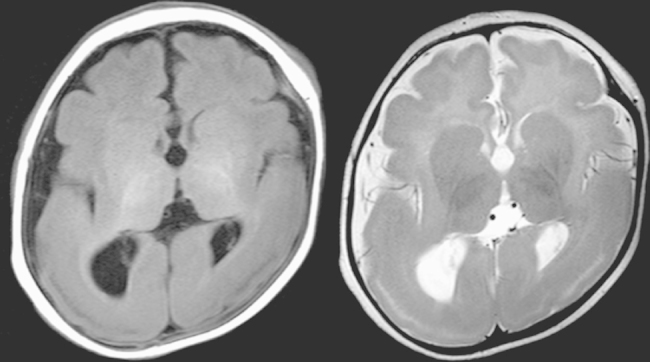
Brain magnetic resonance images of the patient examined at 5 months. T1- (left) and T2- (right) weighted axial images show volume loss and hypomorphic convolution of the brain cortex.
